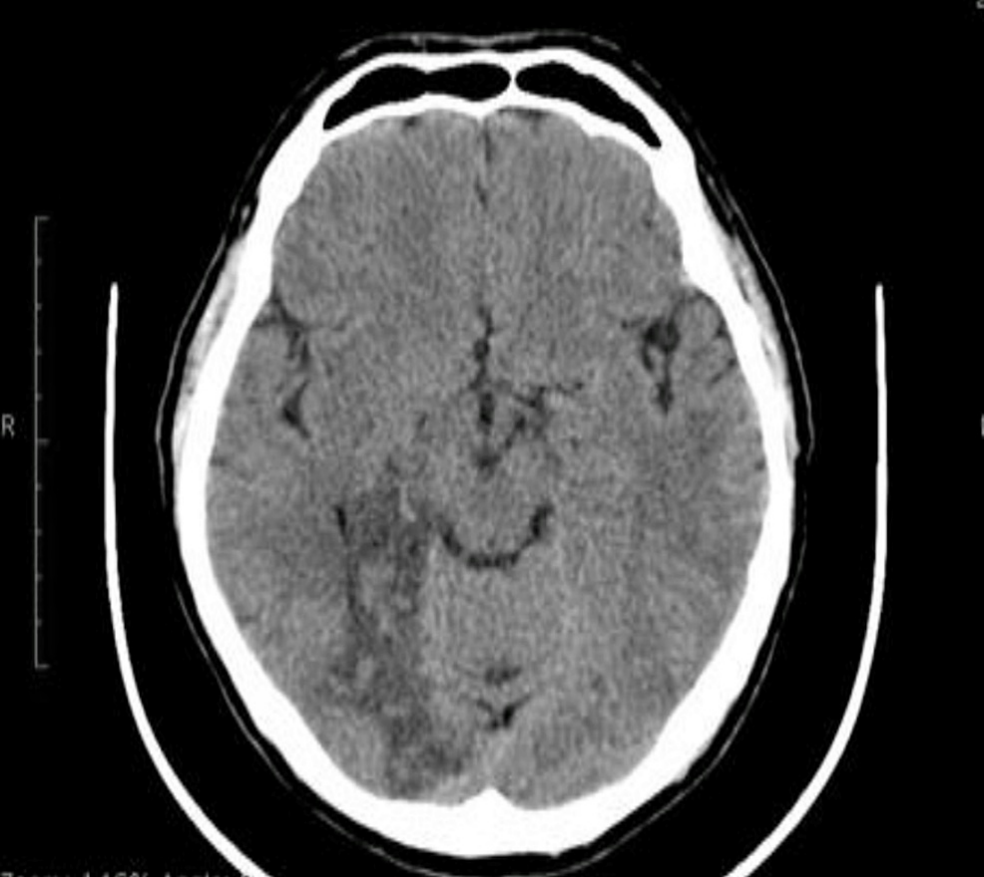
CT scan showing a hypodense region on the right parietal-temporal-occipital region.
